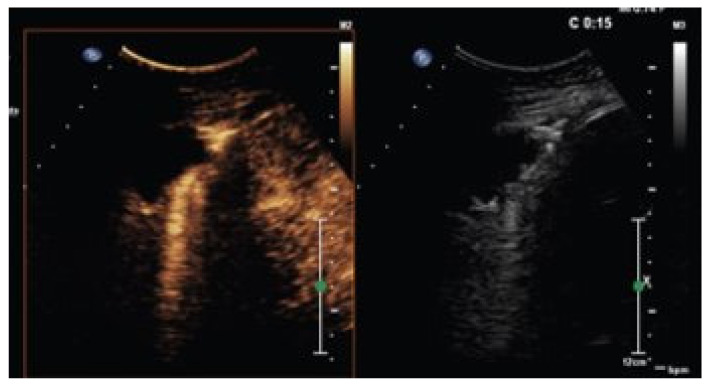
Contrast-enhanced ultrasound findings consistent with peripheral pulmonary infarction. Contrast-enhanced ultrasound (left) and corresponding B-mode ultrasound (right) images demonstrate a peripheral, wedge-shaped subpleural consolidation at the right lung base, characterized by absent contrast enhancement, consistent with pulmonary infarction.
